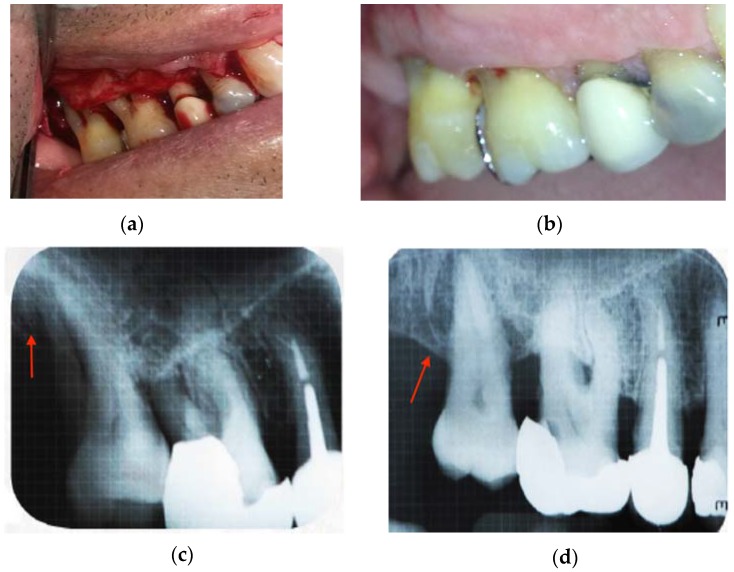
X-rays of one patient with local chronic periodontitis, at the onset of the CH/HAP implant and at the end of the treatment (12 months). The arrows show the alveolar bone growth (c) compared to the oral defect before the implant of the biomaterial (d). In (a) a considerable loss of mucous tissue is observed in the oral defect. In (b) a repair of mucous tissue on the implanted oral defect is observed. CH: Chitosan; HAP: Hydroxyapatite.
